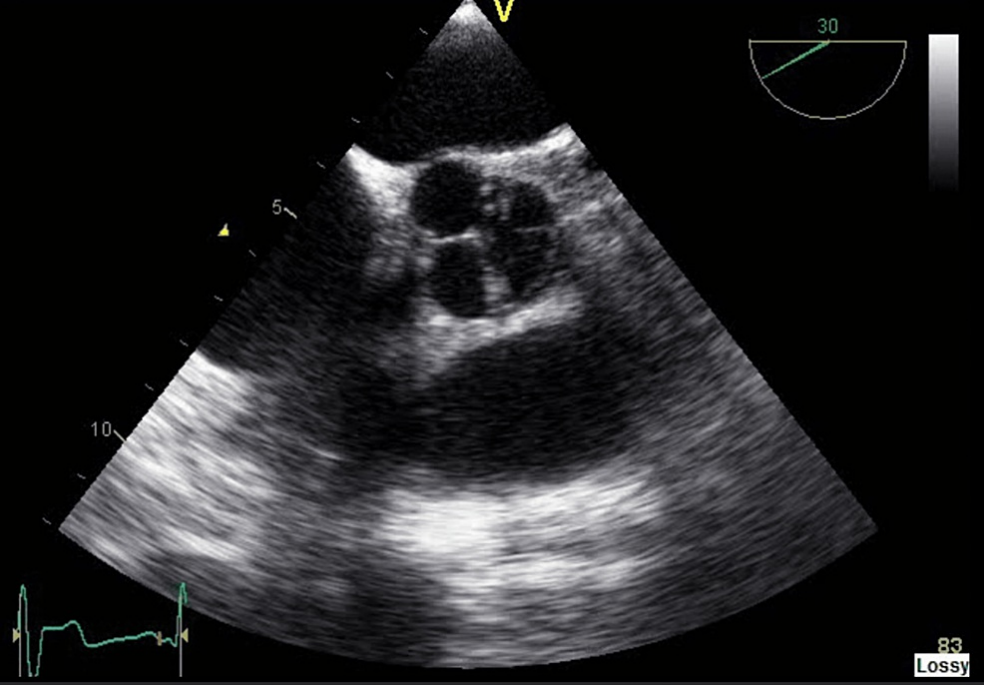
Quadricuspid aortic valve on transesophageal echocardiogram (TEE) short axis view.
